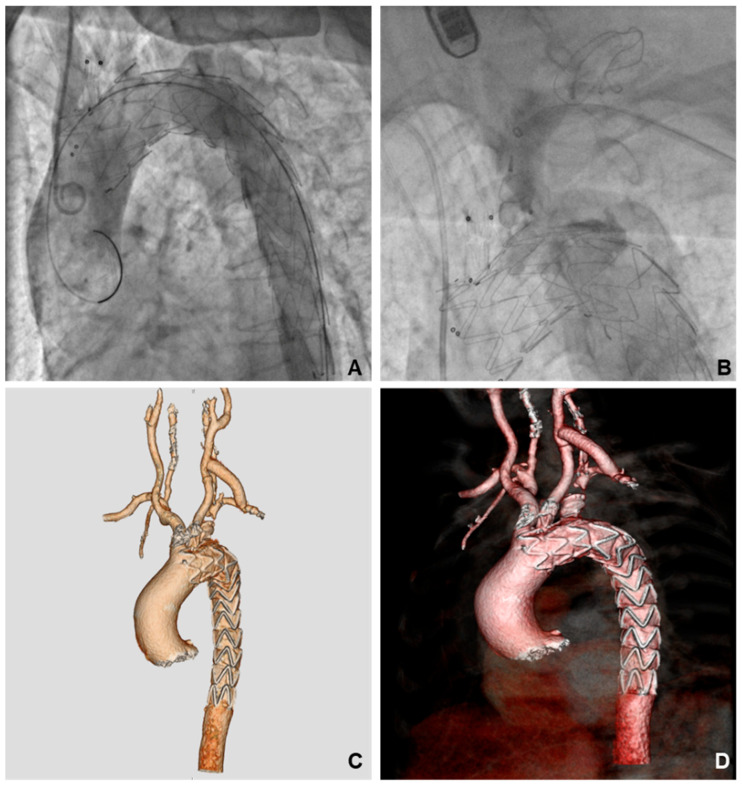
(A) Angiographic control after graft release in landing zone 1 with the branch in the left common carotid artery. (B) Angiography during plug deployment in left subclavian artery. (C,D) Post-operative three-dimensional volume rendering computed tomography.
